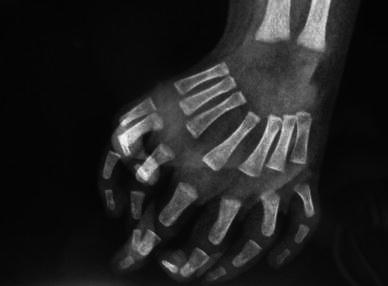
Radiograph of hand shows seven triphalangeal digits with seven corresponding metacarpals all in one plane
